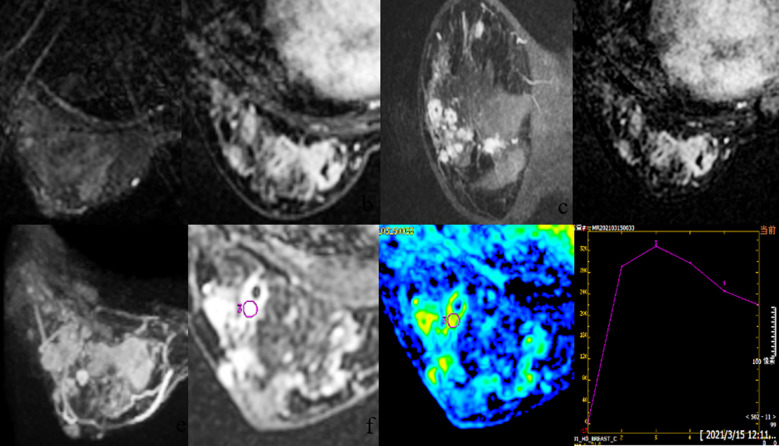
A35-year-old lady had “breast hyperplasia” with dilated mammary ducts (BI-RADS level 4a) On ultrasonography. MRI revealed numerous irregular cystic nodules in the left breast, one of which was approximately 2.5 cm in diameter, isointensity on T2WI (1a), heterogeneous strong signal on DWI (1f), and ADC 2.08 10-3 mm2/s (1 g). Axial contrastenhanced picture of a breast with clustered annular enhancement (1b-c), and the silhouette highlighting the lesion (1d). (MIP image 1e) indicates increased edema of the left breast surface as well as thickening of the blood supply artery. GLM findings were verified by pathological findings (left breast).
